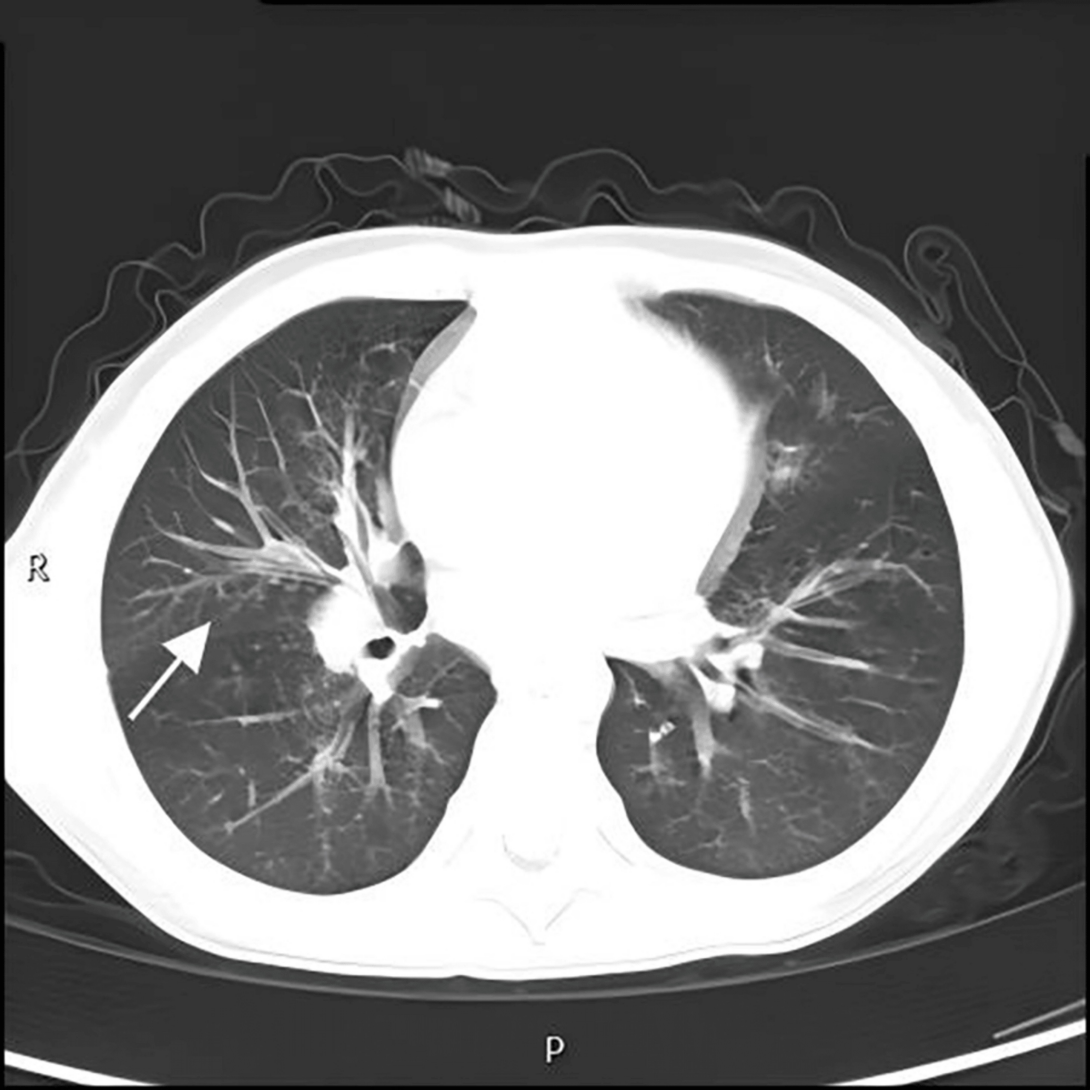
Initial chest radiograph obtained on admission revealing characteristic bronchopneumonia features.
